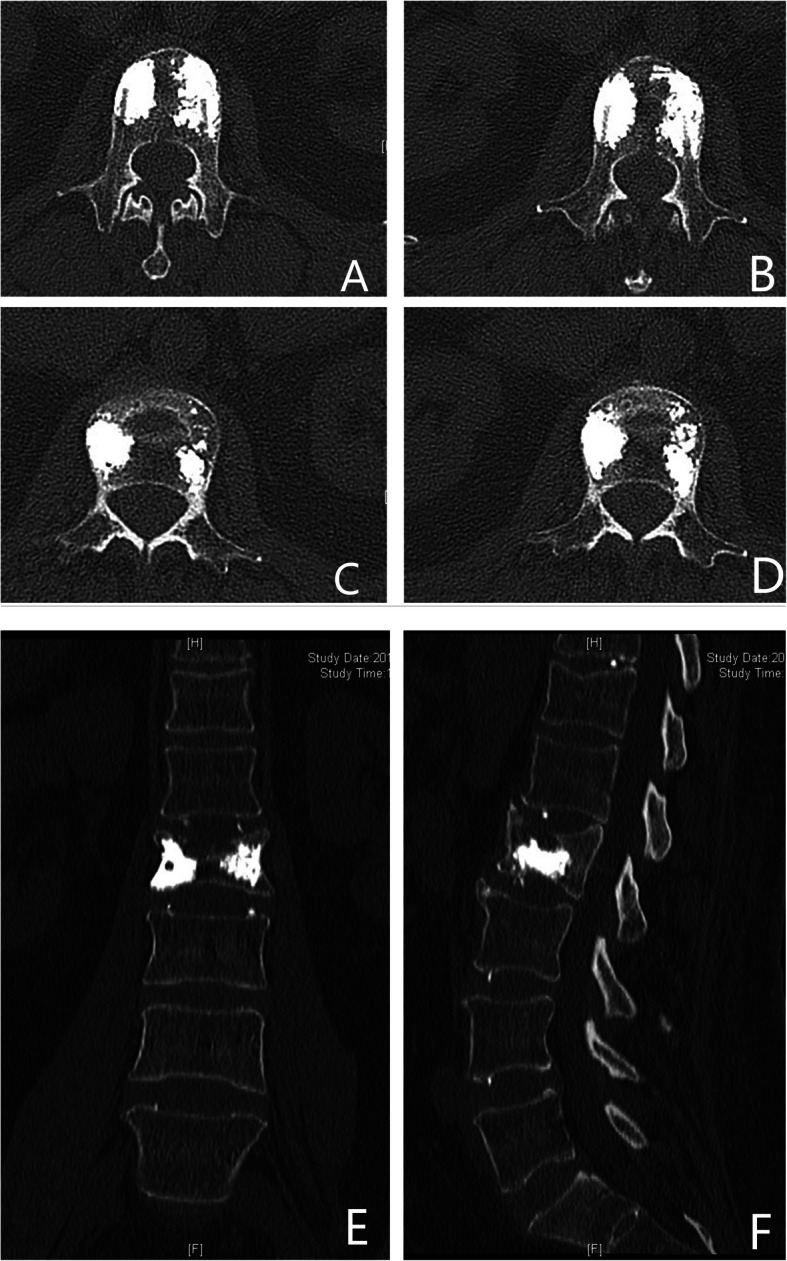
Axial CT radiography showed polymethylmethacrylate (PMMA) filled around the profile of the SNs in an eggshell shape (a–d). Anteroposterior and sagittal CT showed PMMA filled the profile of the SNs in L2 around SN without cement leakage at 1 month
